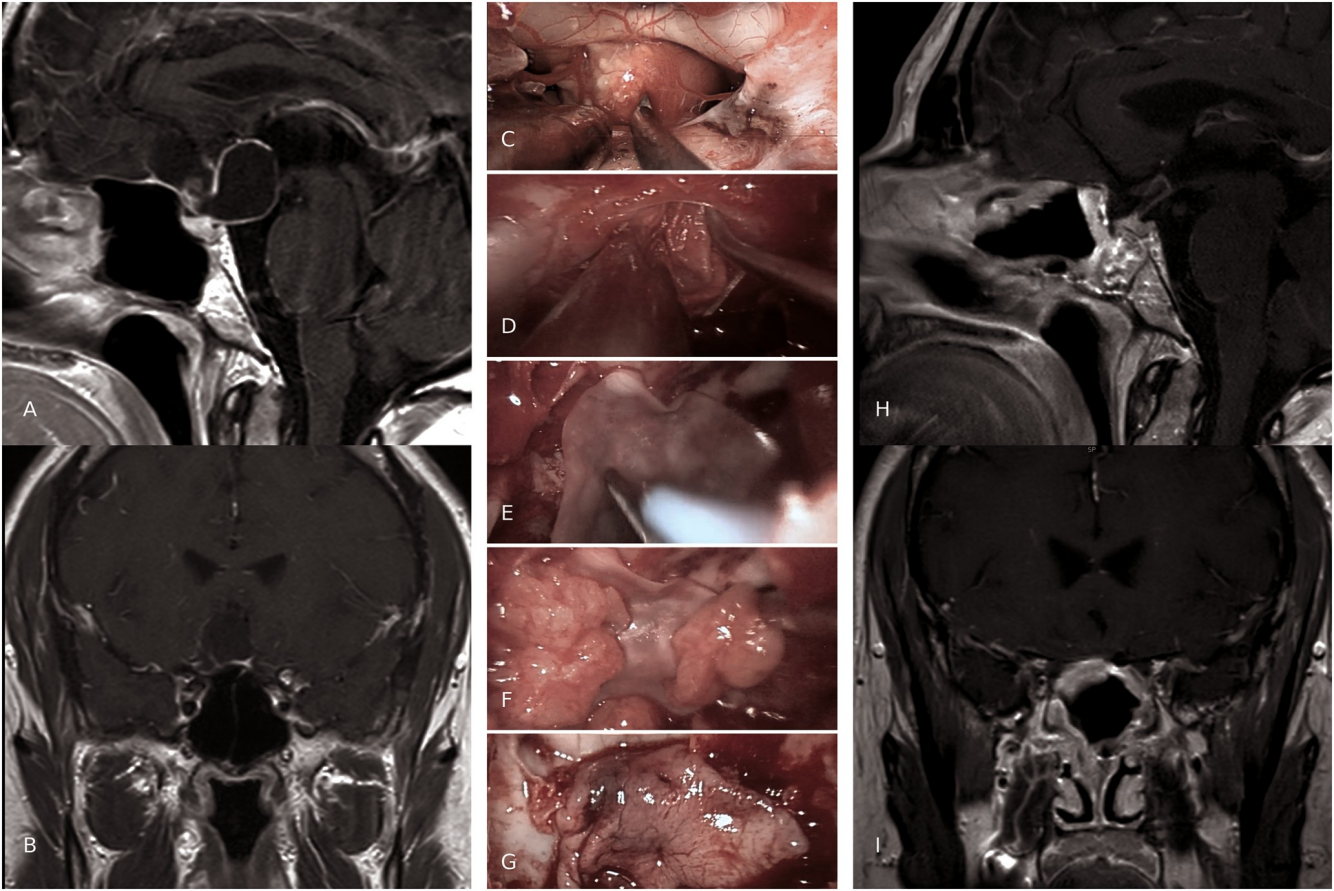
A case example of "sandwich” multilayered reconstruction. Preoperative sagittal (A) and coronal (B) MRI scans, show a T1w hypointense suprasellar 37x25 mm lesion with ring enhancement. C-G intra-operative views of reconstruction steps after a craniopharyngioma removal. C, Transtuberculum-transplanum approach for sopra-sellar pathology; note the optic chiasm pushed forward by the tumor; D, “inlay” synthetic dural substitute; E, "onlay” synthetic dural substitute, which is positioned over a first layer of autologous fat graft; F, pieces of autologous fat graft; G, NSF is fashioned over the layers previously described; three-months post-operative sagittal (H) and coronal (I) MRI scans (T1w with gadolinium), show GTR and the multiple layers adequately placed. No postoperative CSF-leaks was described in the current case.
